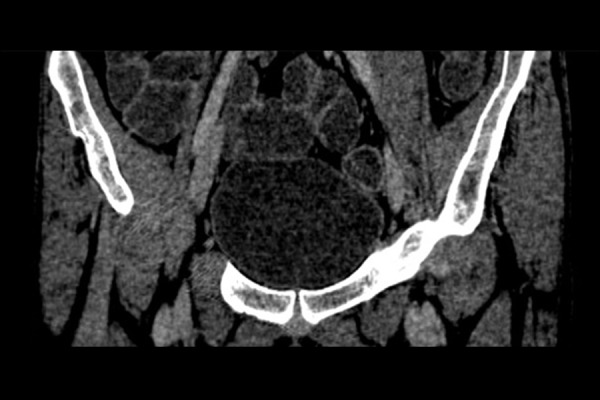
Coronal post contrast CT image showing no evidence of any enhancing focal wall thickening in the fundic region of urinary bladder.
